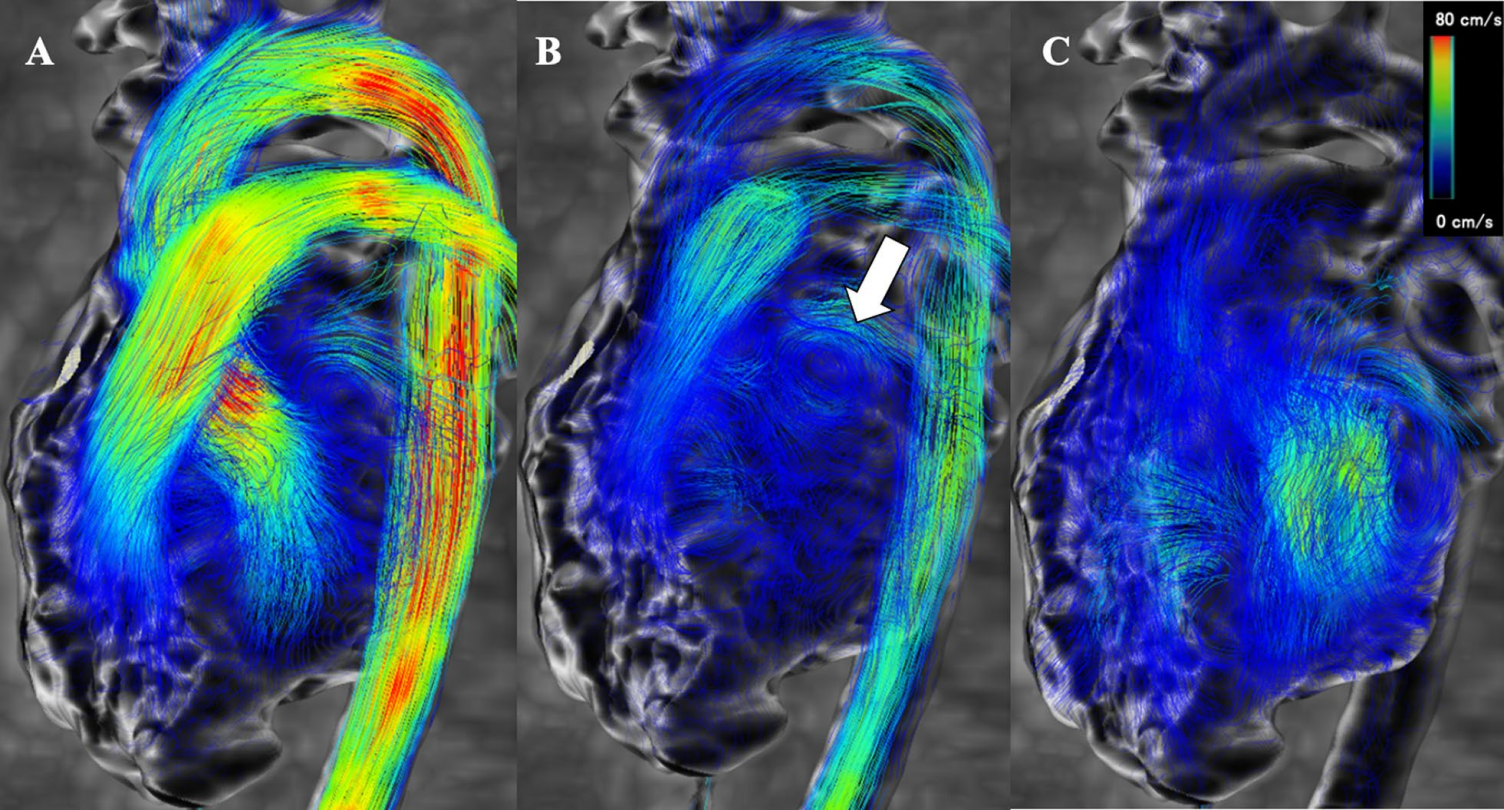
MRI images depicting left atrial vortex flow throughout a single cardiac cycle in a healthy individual are presented. A Pulmonary venous blood enters the left atrium in the early phase of ventricular systole, B Left atrial vortex flow (white arrow) transpires in the late phase of ventricular systole, C Blood flows into the left ventricle during the early phase of ventricular diastole
